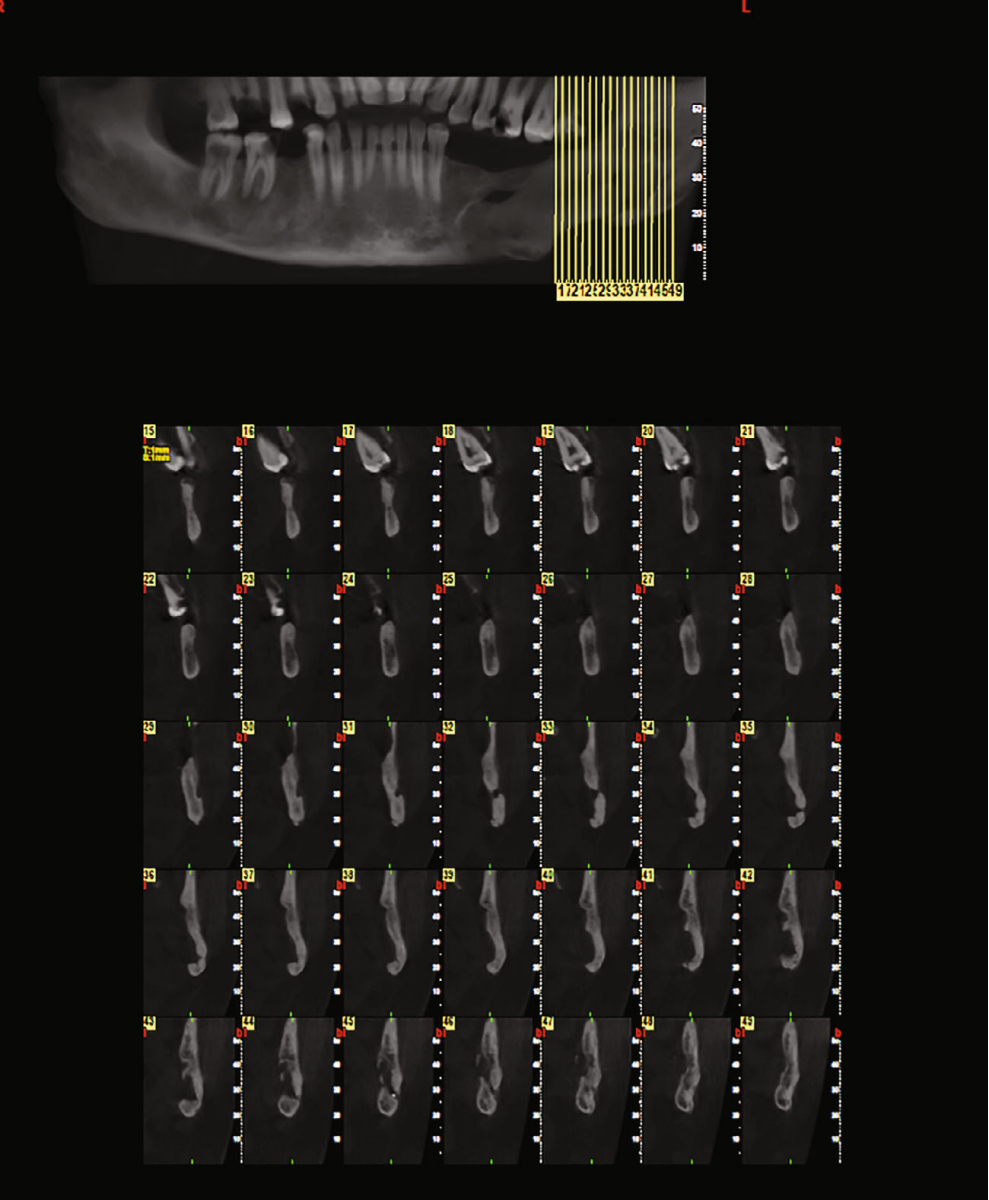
Cross-sectional CBCT images at 3 years postoperatively, indicating spontaneous bone fill of the defect (after ensuring complete spontaneous bone fill of the defect, the plate was removed in another surgical procedure).
